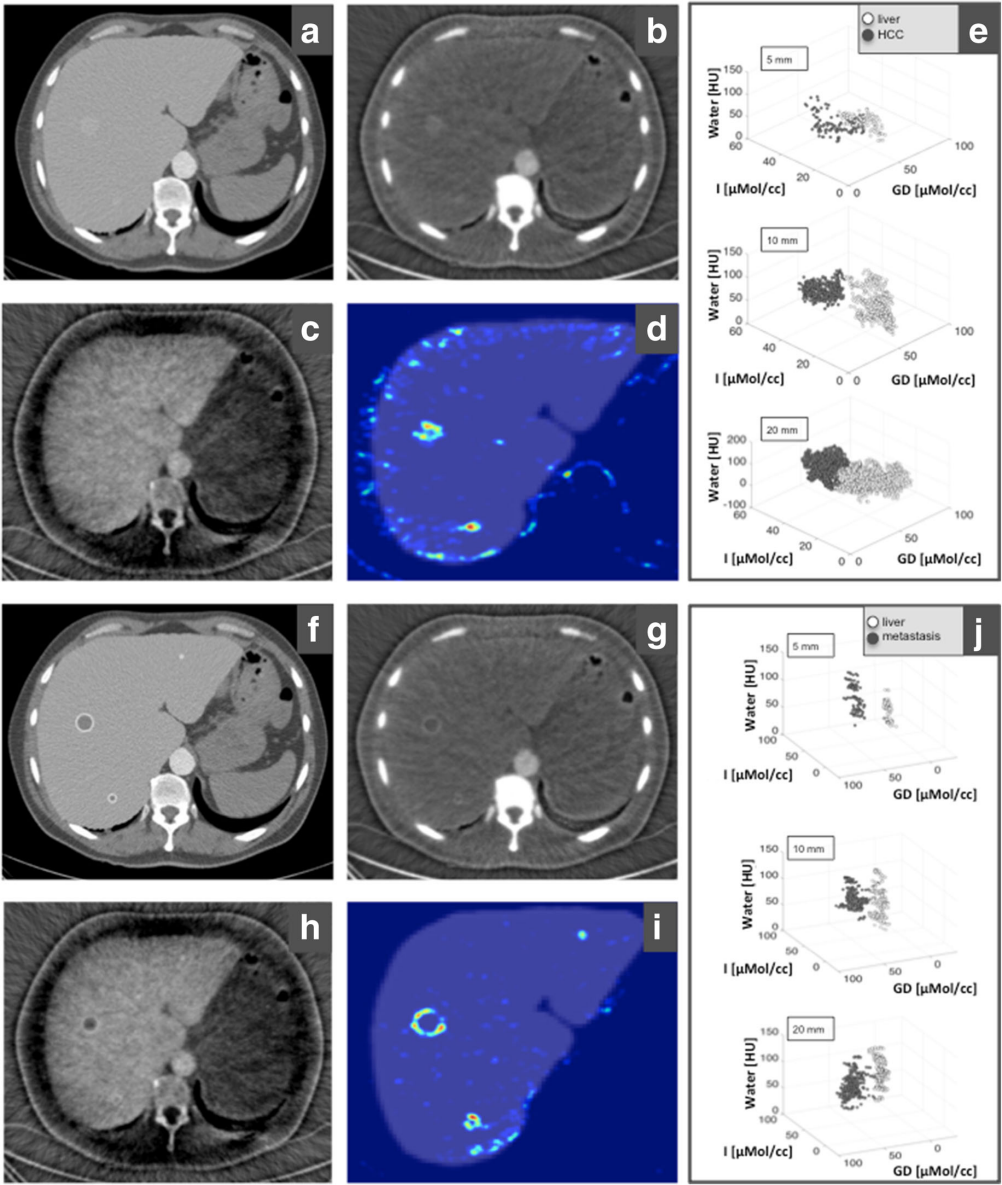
SPCCT imaging of malignant liver lesions, exemplified by HCC (a–e) and metastasis (f–j). a, f Conventional CT image. b Iodine-based image, showing HCC with a typical hyper-enhancement in arterial phase. c Gadolinium-based image of HCC in portal venous phase, which shows only subtle early washout with hypodense presentation in the largest lesion. g, h Iodine- and gadolinium-based image of metastases, with a typical ring-like enhancement in the arterial phase (g), increased in the portal venous phase (h). d
